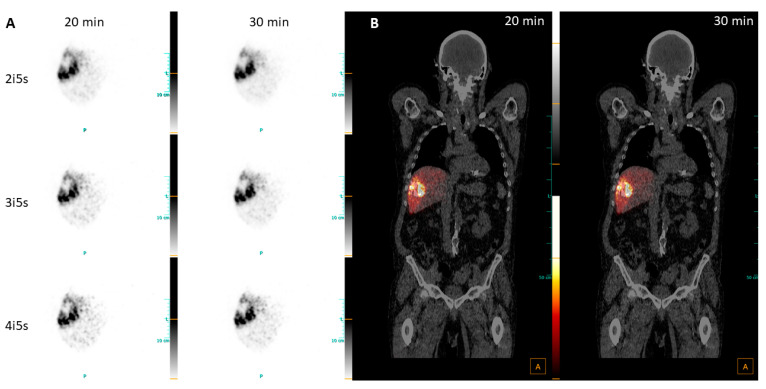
(A) Transversal views of PET images of patient 5, diagnosed with HCC, reconstructed with 2i5s; (B) fused coronal PET/CT images, reconstructed with 2i5s.
